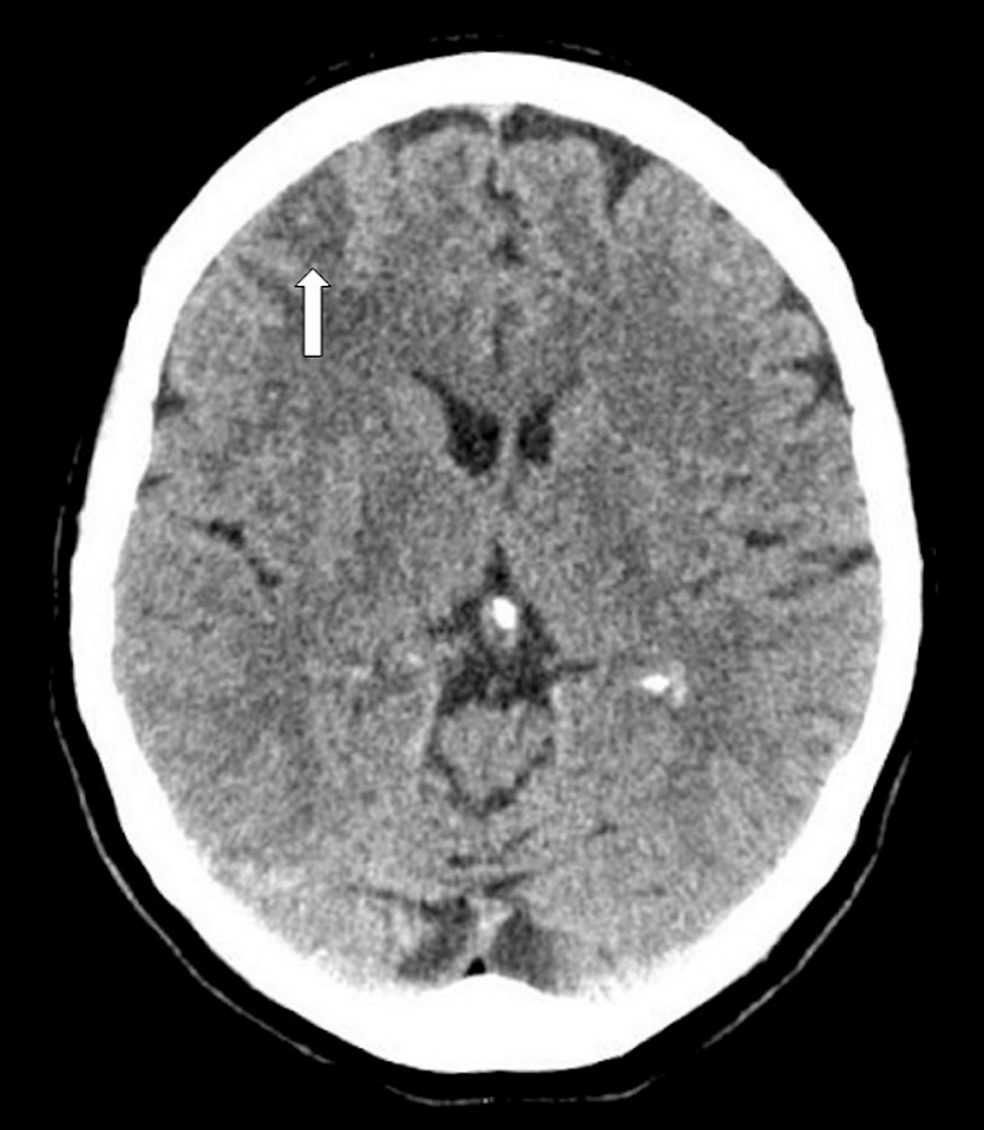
Axial CT image two weeks following the first presentation documented resorption of the blood and a progressive perifocal edema
